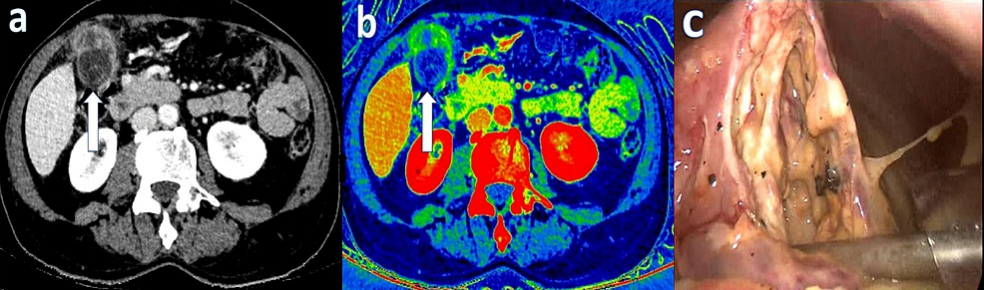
Dual-energy IV contrast abdominopelvic CT performed on a 66-year-old male admitted to the hospital with a complaint of pain in the right upper quadrant revealed perforation of the gallbladder wall. Transmural loss of wall enhancement on (a) 45 keV virtual monoenergetic images (white arrow) and (b) iodine overlay map images (white arrow). (c) Necrosis and perforation in the gallbladder wall.
